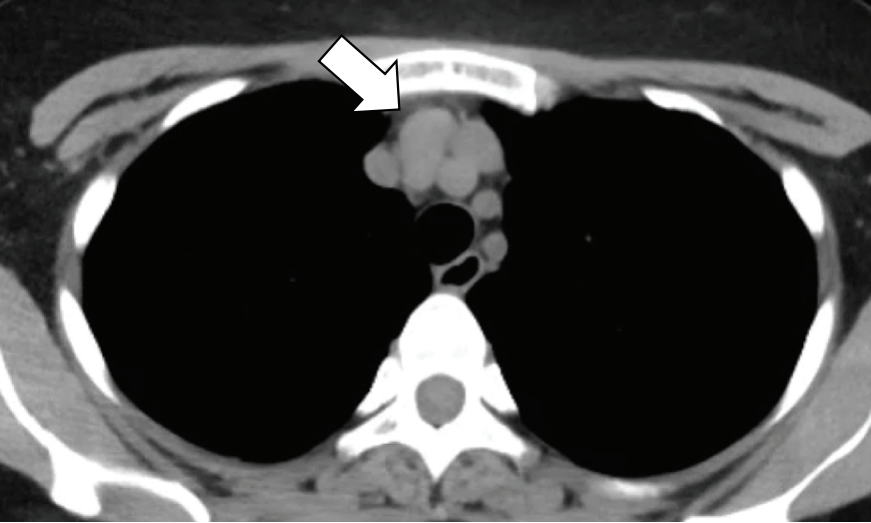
Chest CT showing a 2.5 × 1.5-cm nodule (white arrow) located between the right brachiocephalic vein and brachiocephalic artery in the upper mediastinum.
